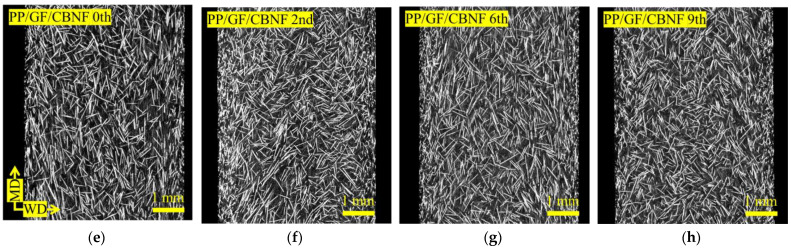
X-ray CT images of the skin region. Images (a–d) correspond to PP/GF, and images (e–h) to PP/GF/CBNF, after 0, 2, 6, and 9 extrusion cycles.
